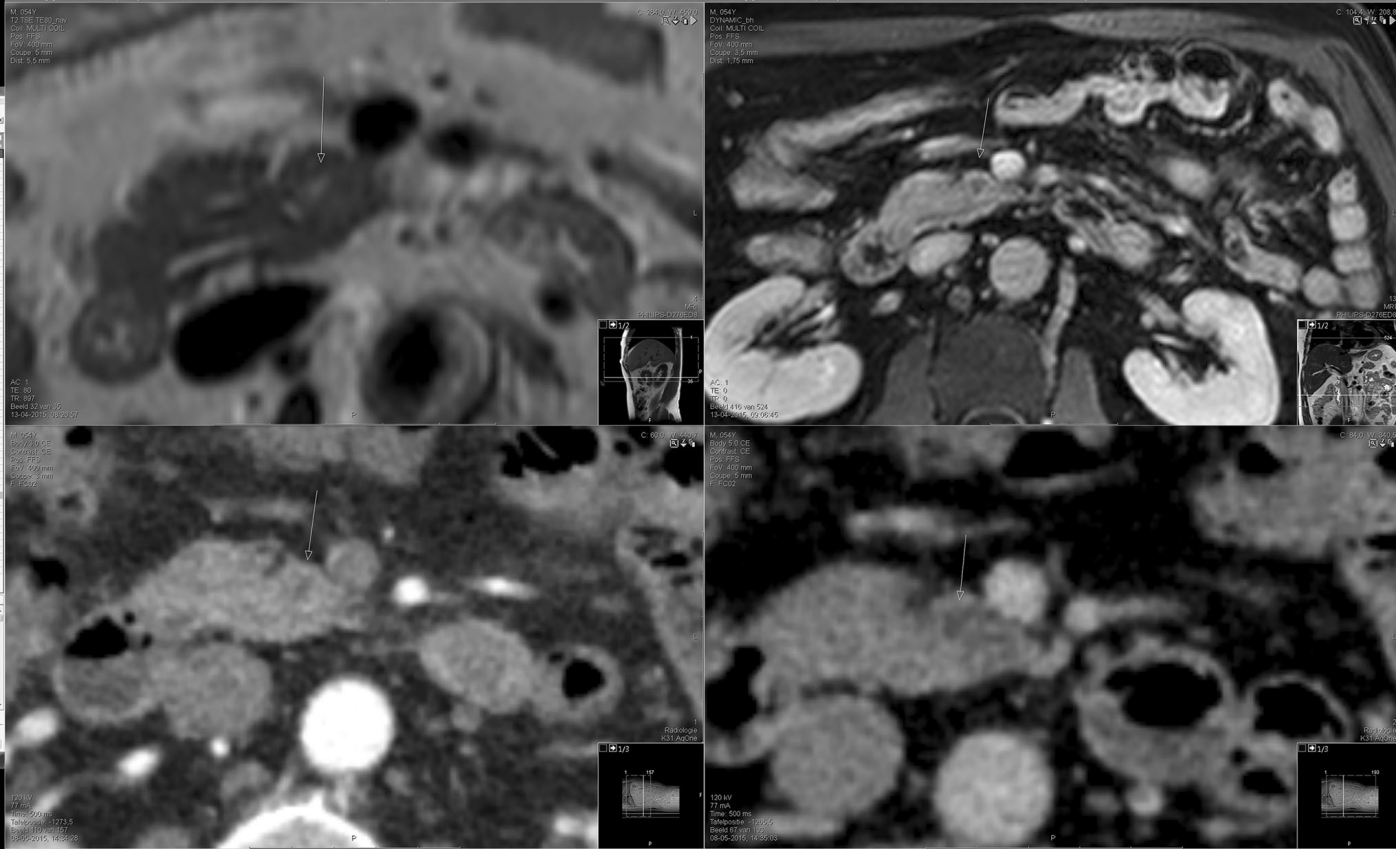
MRI (*upper panel*) and CT-scan (*lower panel*) of the pancreas in case 1
